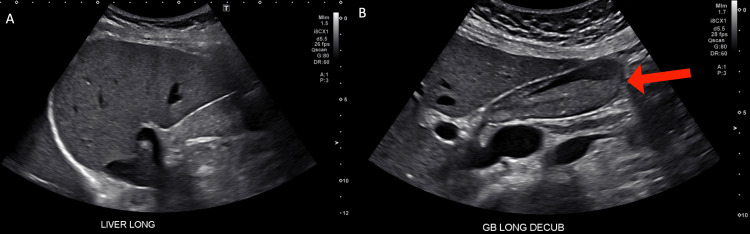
Normal abdominal ultrasound of the patient. The abdominal ultrasound images show (A) the long axis of the liver with no significant pathologies and (B) the long axis of the gallbladder (red arrow) with cholelithiasis without evidence of cholecystitis.
